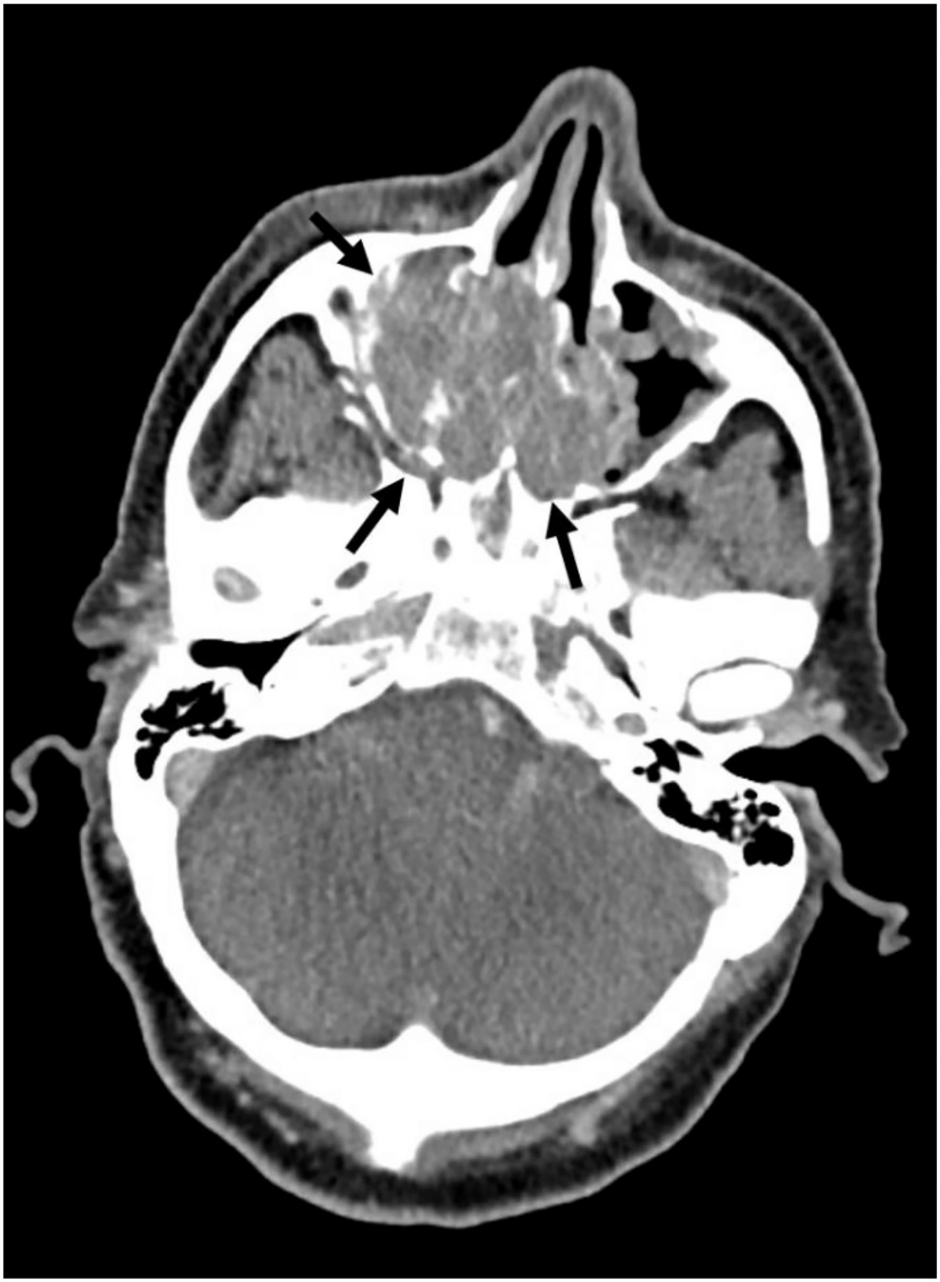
CT image of primary sinonasal SDC tumor (indicated by black arrows).
